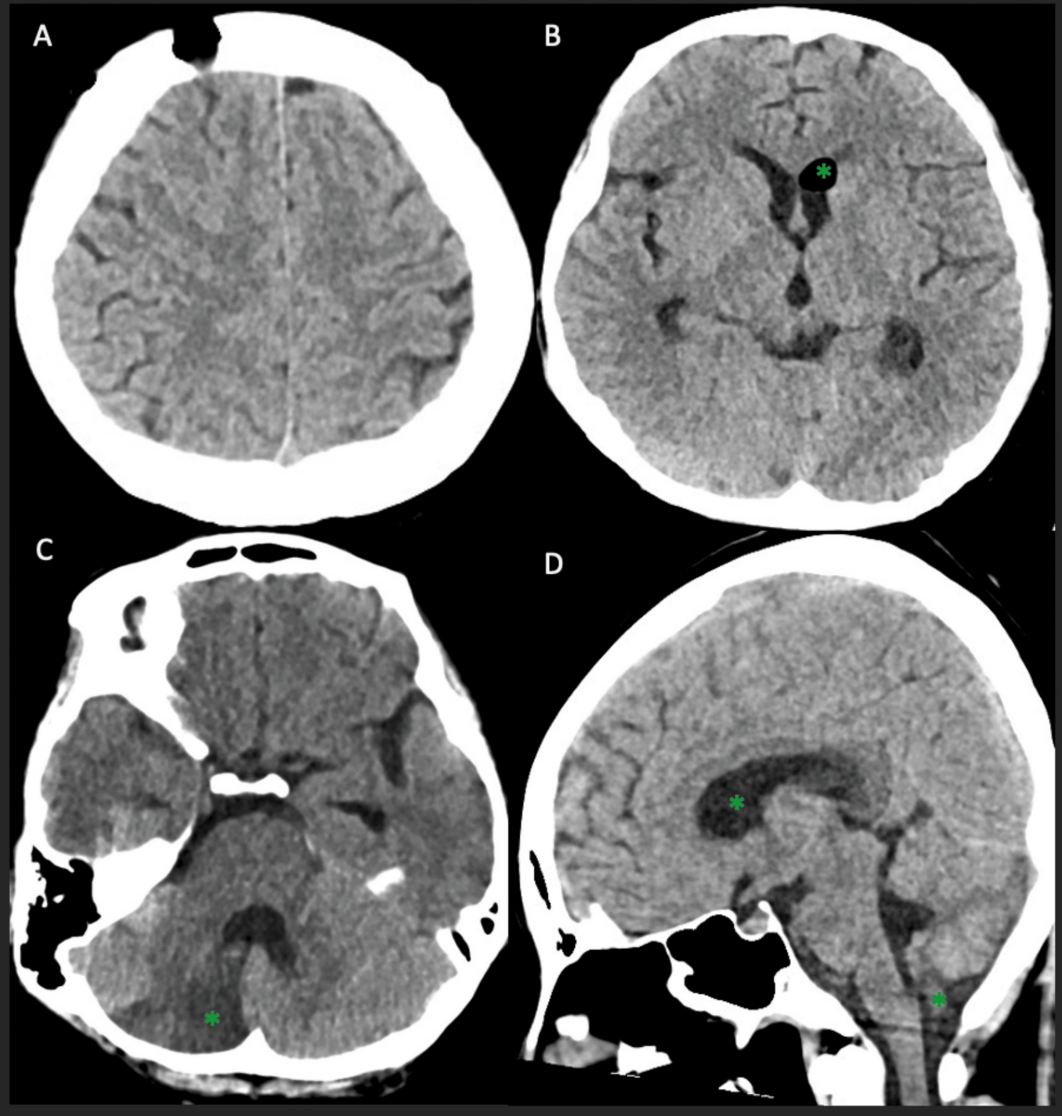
Follow-up CT of the brain prior to discharge (A-D) Established right cerebellar infarct in the posterior inferior cerebellar artery (PICA) territory, with interval resolution of the previously noted right frontal subarachnoid hemorrhage, obstructive hydrocephalus, posterior fossa edema, and tonsillar descent. Interval development of a small air pocket within the frontal horn of the left lateral ventricle is noted, consistent with post-procedural change (green asterisks).
